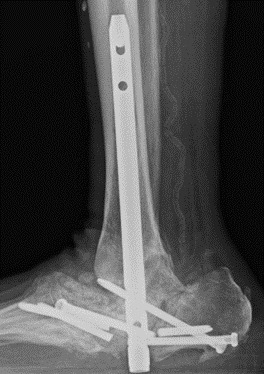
Case 3 lateral ankle radiograph showing nonunion and hardware failure after tibiotalar arthrodesis.
